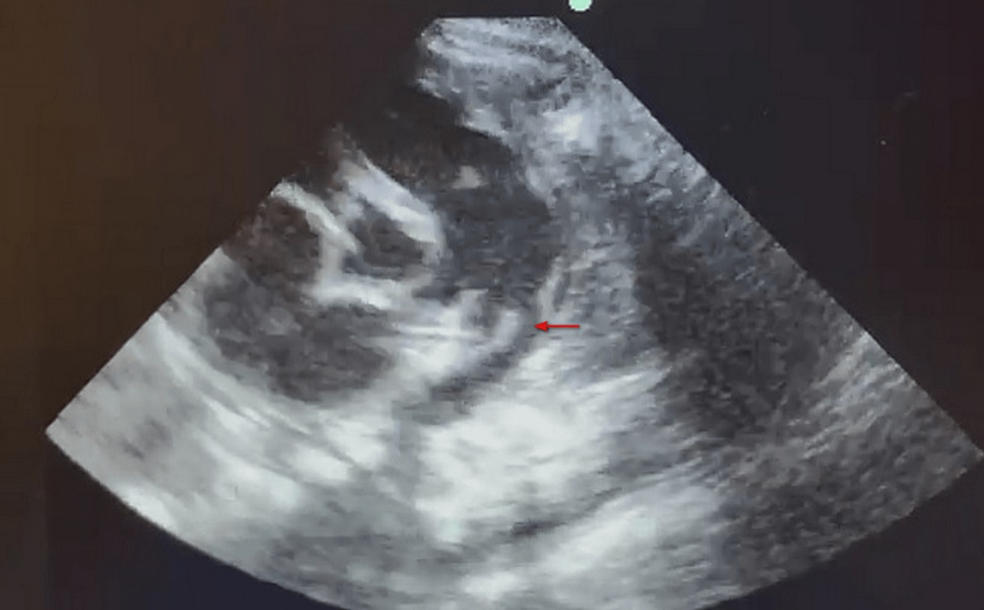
Two-dimensional transthoracic echocardiogram showing the patent ductus arteriosus. The echocardiogram showing the patent ductus arteriosus measuring 3.7 mm, as seen in the parasternal short axis view marked by a red arrow.
